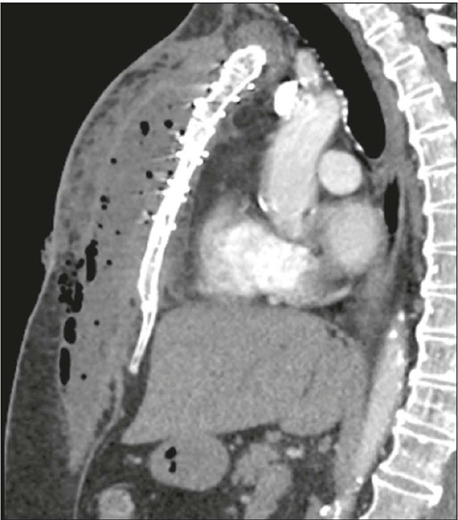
Superficial infection of the surgical wound (sagittal CT image;
